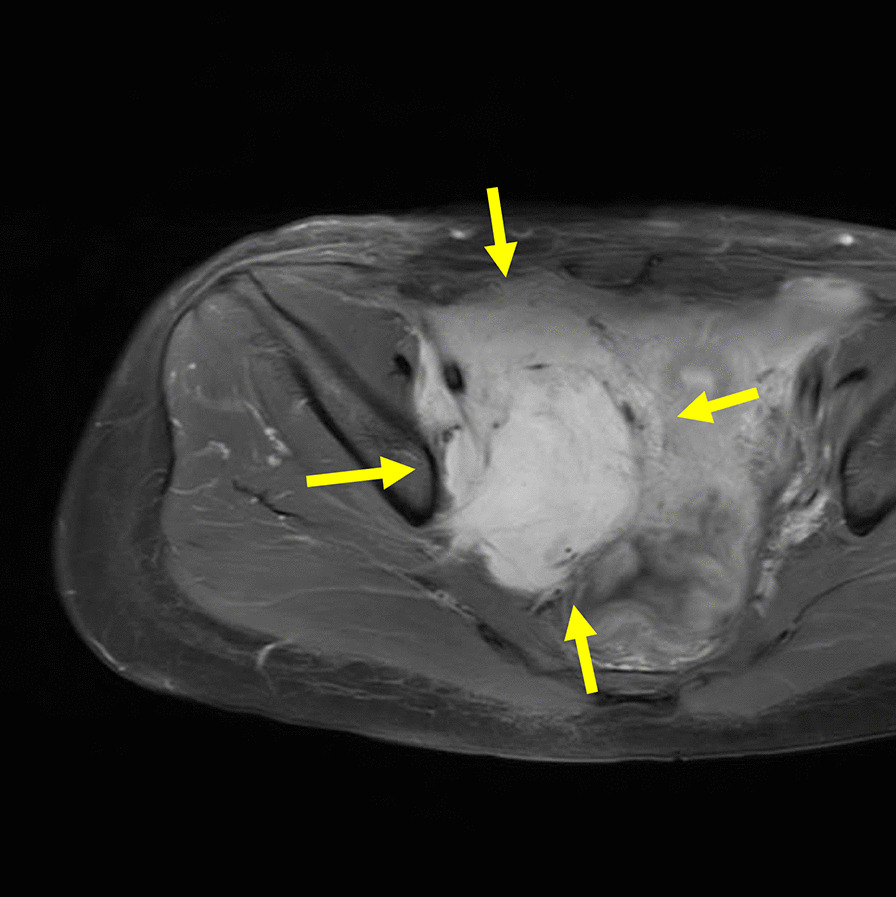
Post-gadolinium axial magnetic resonance imaging of the pelvis showing strong and homogeneous enhancement of lesion by intravenous administration of gadolinium (yellow arrow)
